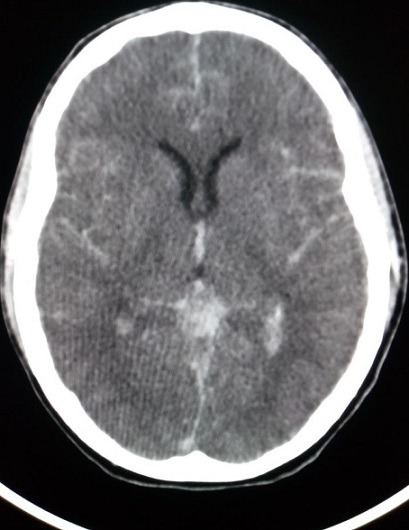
Unenhanced CT axial image shows subarachnoid blood with intra-ventricular extension and peripheral hemorrhage outlining the cerebral sulci
